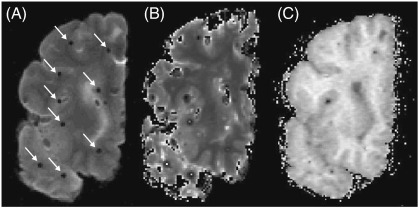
Magnetic resonance imaging of an exemplary post mortem MS brain slice. (A) T_2_ weighted image, (B) T_1_ relaxation map, (C) magnetisation transfer ratio (MTR) map. Arrows indicate artefacts due to tooth picks with which tissue was fixed to a cork board.
